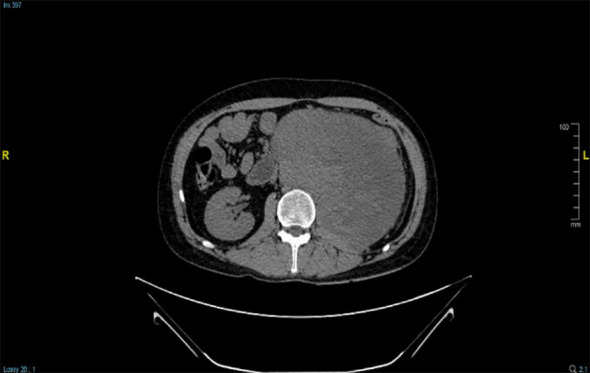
Enhanced CT showing retroperitoneal rhabdomyosarcoma.
